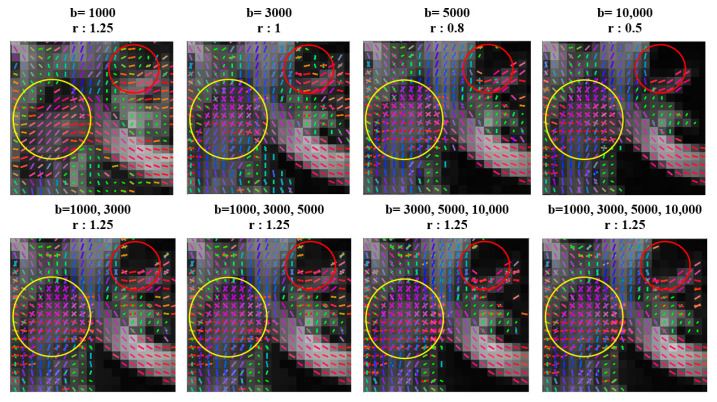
Effect of sampling schemes on fiber-tracking results at crossing regions (e.g., corpus callosum marked by yellow circles). Red circles show regions including false-positive fibers. The optimal values of the diffusion sampling length ratio (r) were determined for each DWI scheme using the optimization procedure specified in DSI Studio.
